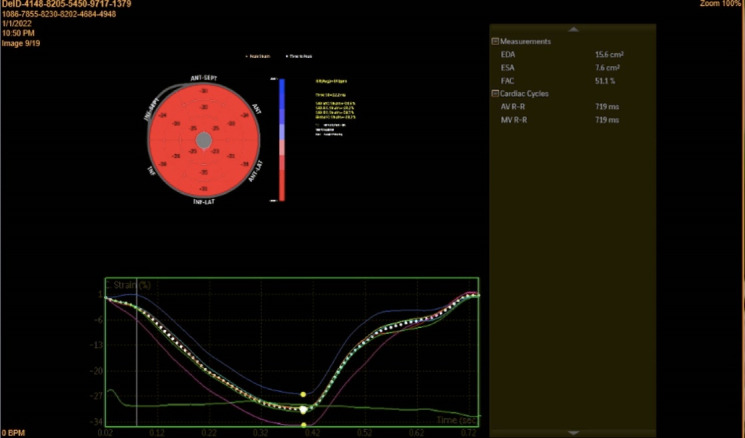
Data collection was done by a single skilled echocardiographer. Inter and intraobserver variabilities were tested via random repetitions by the same echocardiographer at 1-month intervals and by a second echocardiographer, who was blind to the first observer’s measurement. Finally, the parameters were compared between subjects with and without dyspnea. The study protocol was approved by the Review Board of cardiology. (IR.TUMS.MEDICINE.REC.1399.977)
